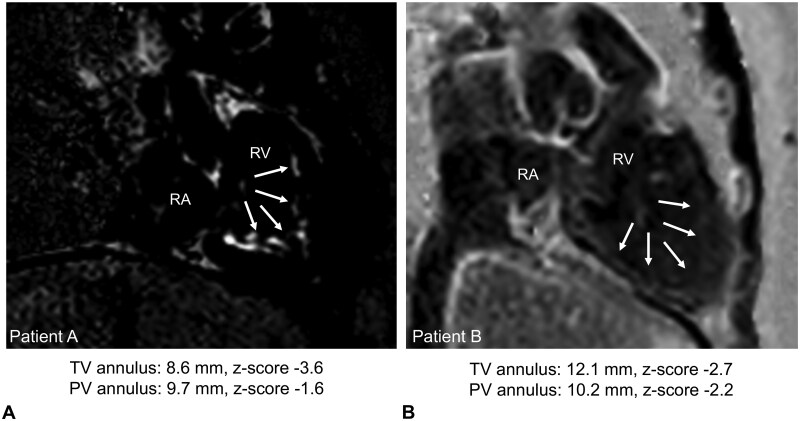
Representative preoperative MRI studies from patients with PA/cPS-IVS. Preoperative MRIs from 2 patients with PA/cPS-IVS demonstrated prominent, confluent subendocardial late gadolinium enhancement throughout the RV cavity (arrows), with an infiltrative growth pattern extending into the underlying myocardium. Corresponding echocardiographic valve measurements are shown below. Abbreviations: PA/cPS-IVS, pulmonary atresia/congenital pulmonary stenosis with intact ventricular septum; RA, right atrium; RV, right ventricle.
